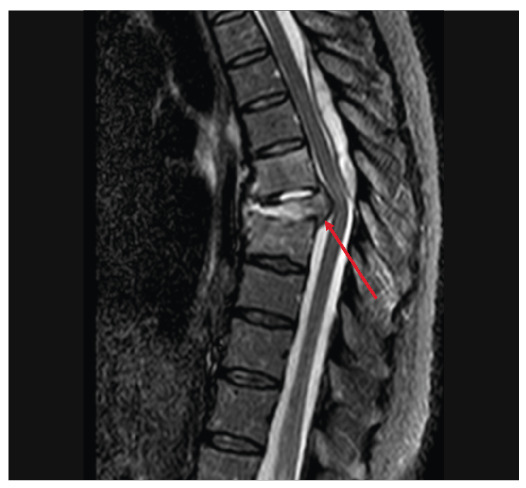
Magnetic resonance imaging. Sagittal T2 short tau inversion recovery showing the hyperintensity of the T6-T7 intervertebral disk and the absence of the internuclear cleft. Hyperintensity of the adjacent vertebral bodies was also observed. The lesion posteriorly compressed the dural sac and the spinal cord (arrow).
